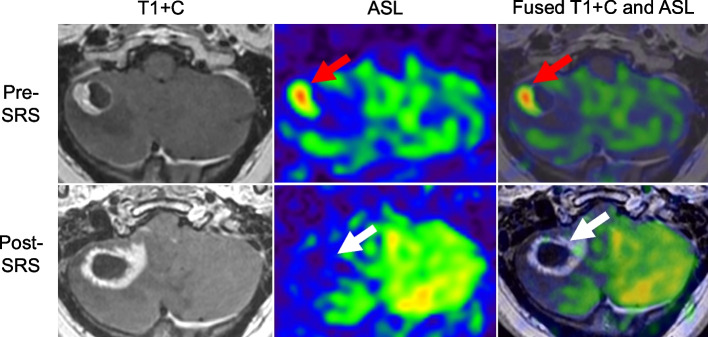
Top row*: A solid and cystic metastasis in the right cerebellum with high blood flow on ASL perfusion and fused post-contrast T1 and ASL images (*red arrows*). *Bottom row*: Enlargement of the cystic lesion with thicker but irregular rind of enhancement, 13 months after initial stereotactic radiosurgery. No elevated blood flow is seen on ASL perfusion and fused post-contrast T1 and ASL images (*white arrows*). Surgical resection of the suspected metastasis performed immediately after the post-stereotactic radiosurgery MRI revealed extensive radiation necrosis and absence of tumor
